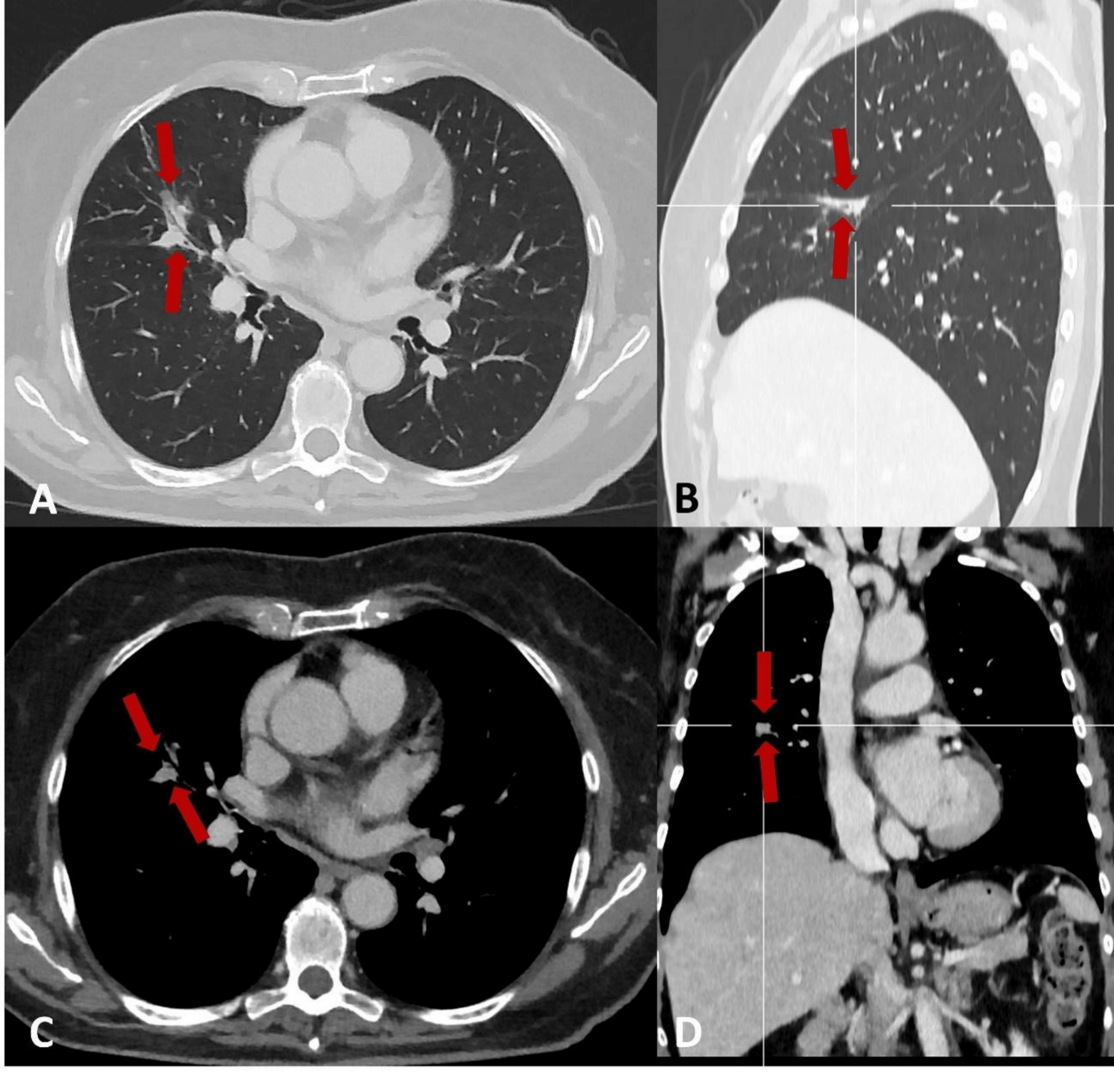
Chest CT showing an ill-defined solid area (red arrow) in the middle lobe. (A) Lung window axial plane. (B) Lung window sagittal plane. (C) Soft tissue window with contrast axial plane. (D) Soft tissue window with contrast coronal plane. CT: computed tomography
